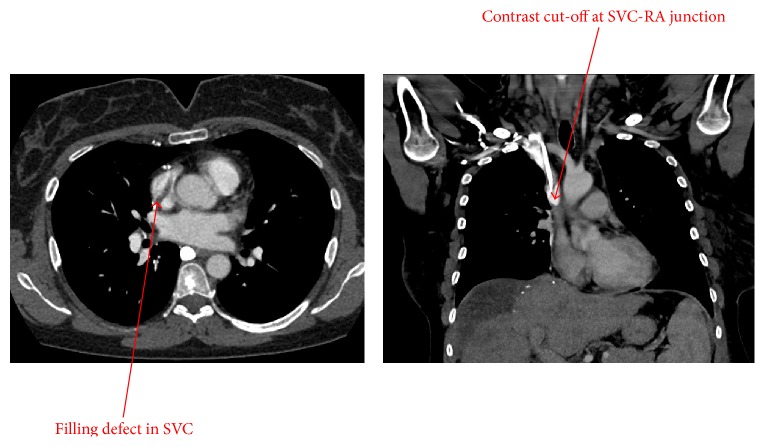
CT angiography of the chest showing a filling defect in the SVC and contrast cut-off at the SVC-RA junction caused by a thrombus.
